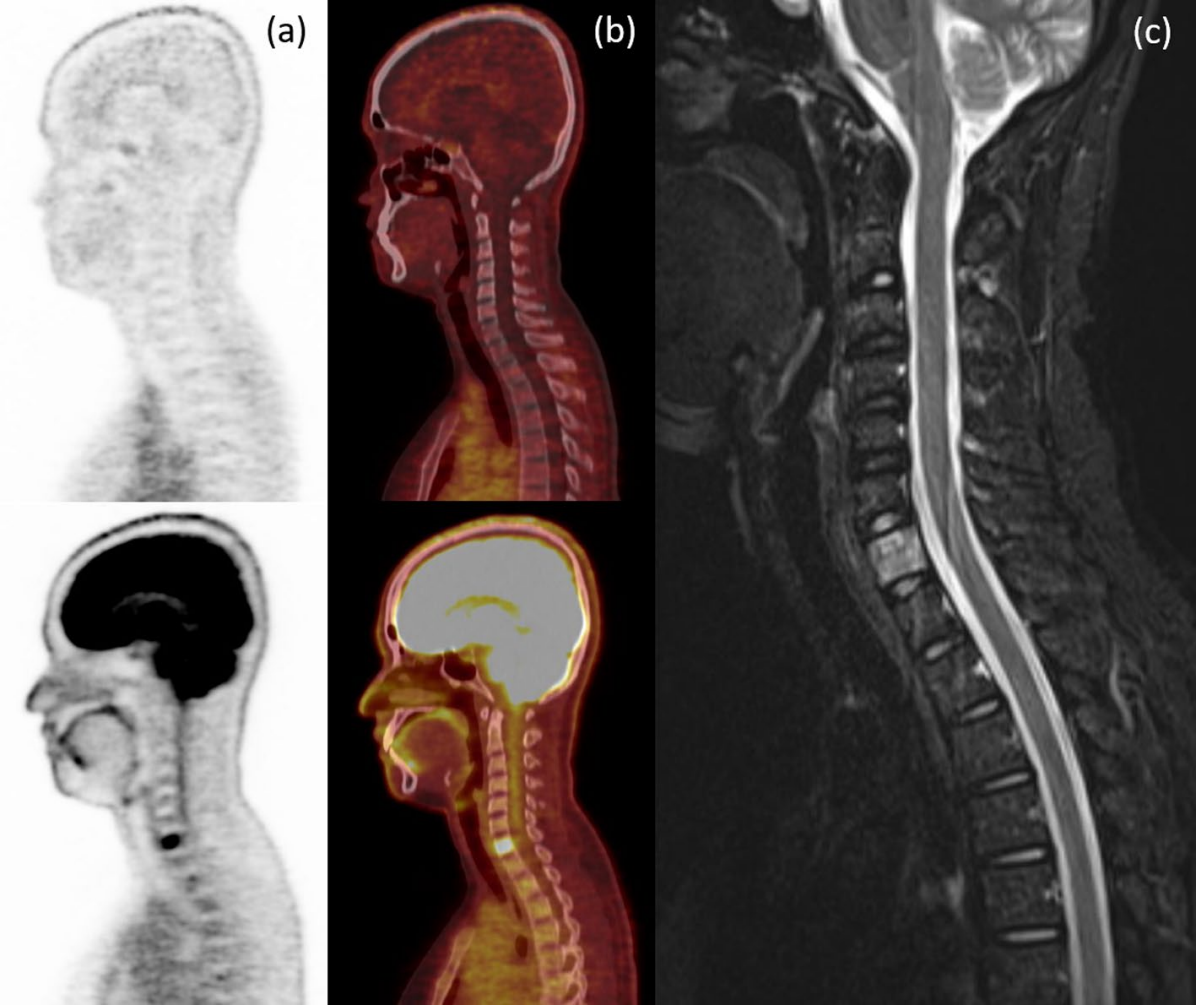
Sagittal PET a and sagittal fusion b images of ^18^F-FES (above) and ^18^F-FDG (below) for ^18^F-FDG-avid hyperT2 bone metastasis at C7 vertebra on MRI (c) without ^18^F-FES avidity
